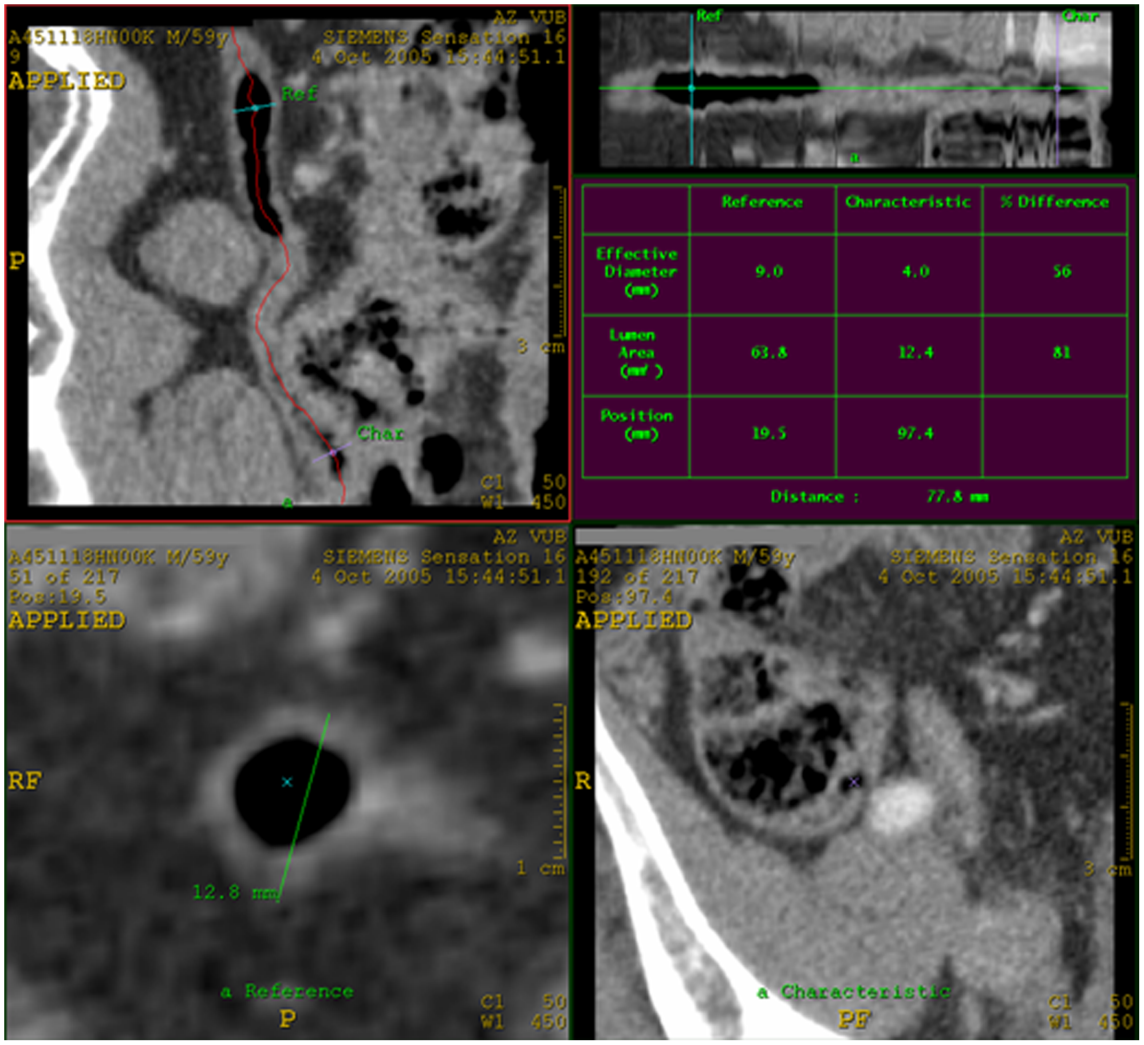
Measurement of maximum outer diameter of the normal appendix on CT. In the top left side viewport the appendix was visualized along its complete length. Here the reader looked for the image truly perpendicular to the axis of the appendix, which corresponded to the maximal outer diameter as seen in the left bottom side viewport. The maximal outer diameter of the appendix was measured in the bottom left side viewport. In the top right side viewport, the appendix was seen as a linear structure.
